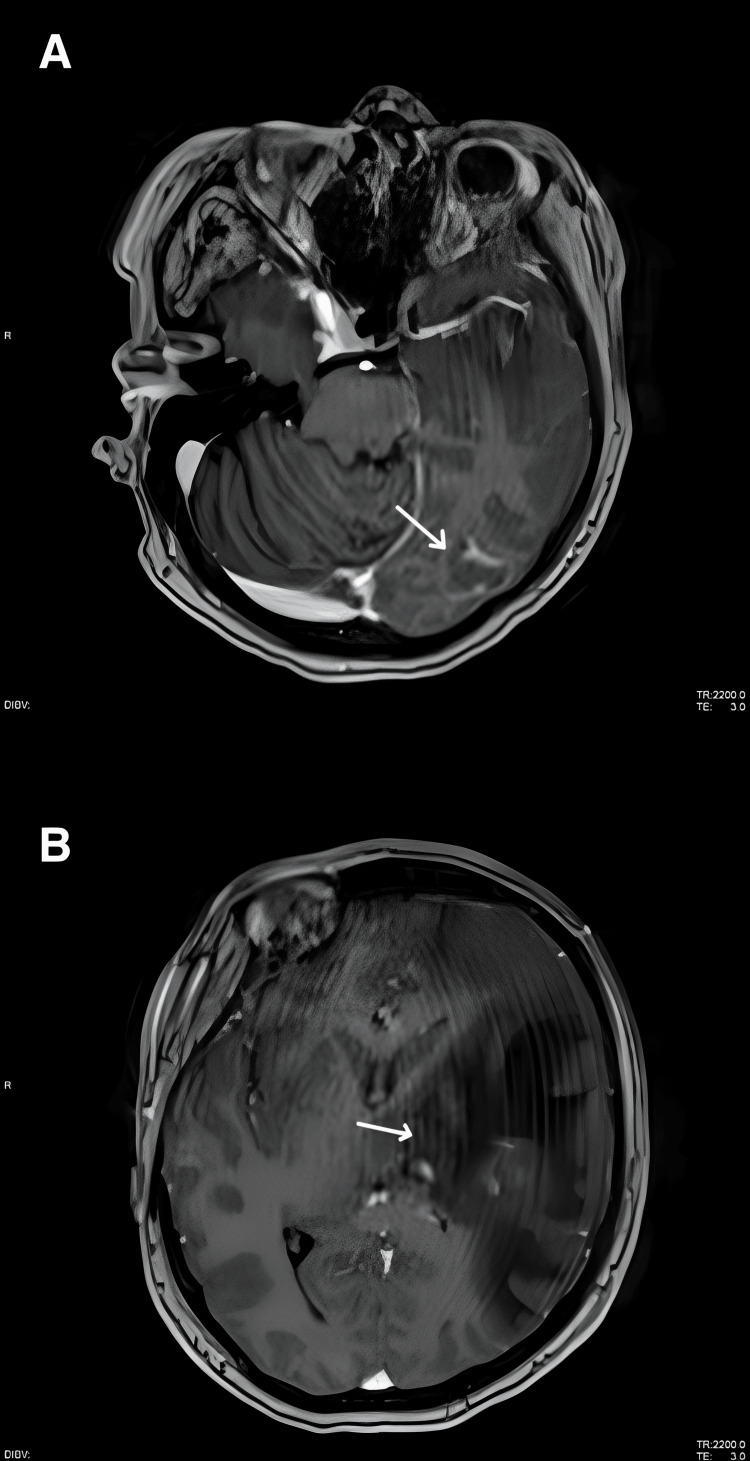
Follow-up contrast-enhanced MRI performed two weeks after treatment initiation (A) Persistent enhancement of the left occipital lesion with minimal interval improvement (white arrow). (B) Increased enhancement of the left thalamic lesion (white arrow), suggestive of progressive disease.
